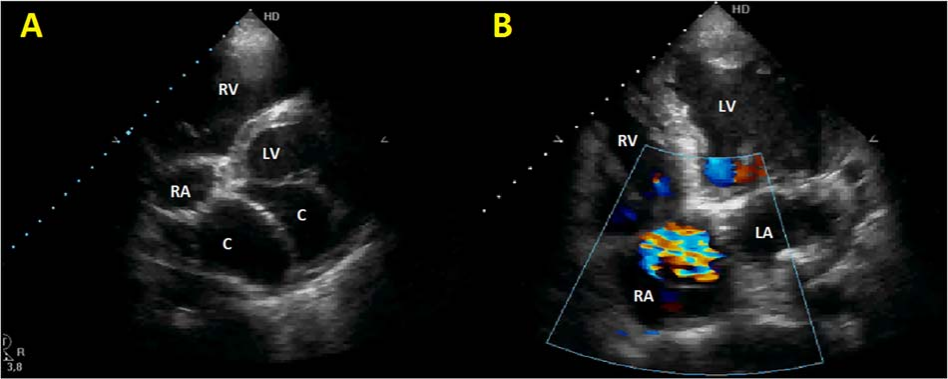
Initial TTE. (A) Parasternal short-axis view showing a bi-atrial cystic lesion; (B) apical 4-chamber view showing color Doppler aliasing in the RA, indicating a shunt. RV, right ventricle; LV, left ventricle; C, cystic lesion.
